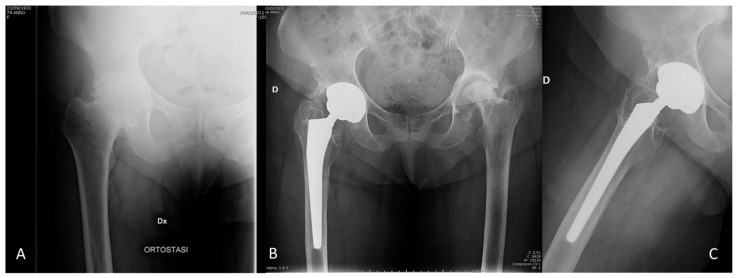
Ten-year radiographic follow-up of a THA performed in a woman aged 79 years with hip osteoarthritis, showing excellent osseointegration around the stem, lack of radiolucent lines, and minimal signs of stress-shielding with slight distal cortical hypertrophy and pedestal formation. (A) Preoperative AP radiograph of the affected hip. (B,C) AP and lateral radiographs at 10-year follow-up. A Brooker 3 heterotopic ossification was detectable laterally inside the capsule. D means right side.
